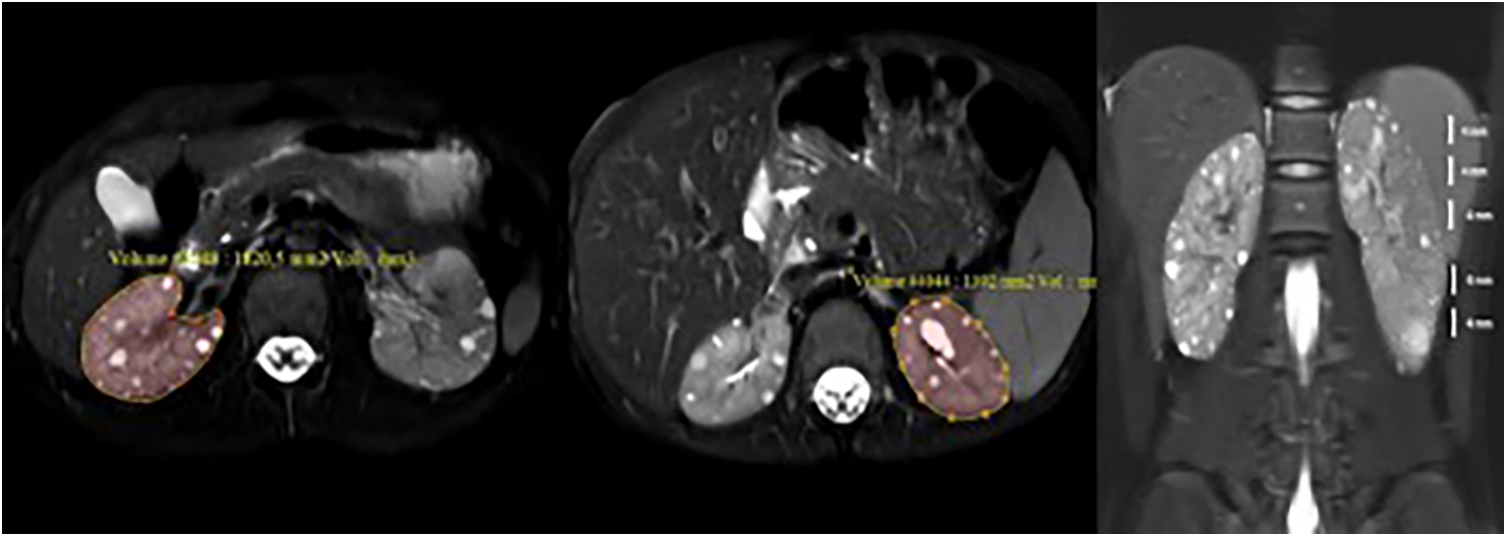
Measurement of kidney volume with MRI. Kidney volumes were determined by meticulously tracing the area on each individual slice of the axial fat-saturated T2-weighted sequence. This process involved calculating the sum of the organ areas present on each slice and then multiplying the sum by the thickness of each slice. In essence, the volume was derived by multiplying the calculated area by the height of the slices (volume = area × height). Subsequently, the calculated volumes of the right and left kidneys were summed to determine the total kidney volume (TKV).
